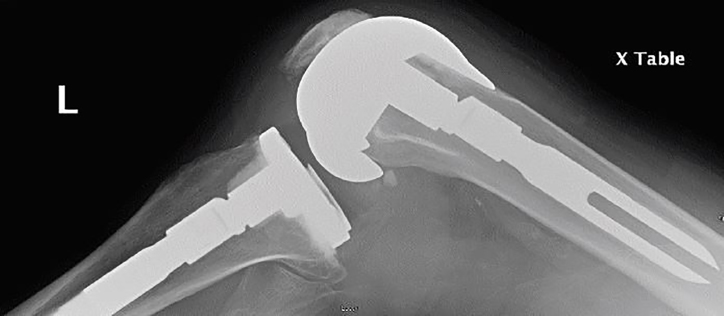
Initial lateral radiograph of cam-jump dislocation of prosthetic knee joint. The femoral component is subtly displaced anteriorly versus the tibial component of the prosthesis.
